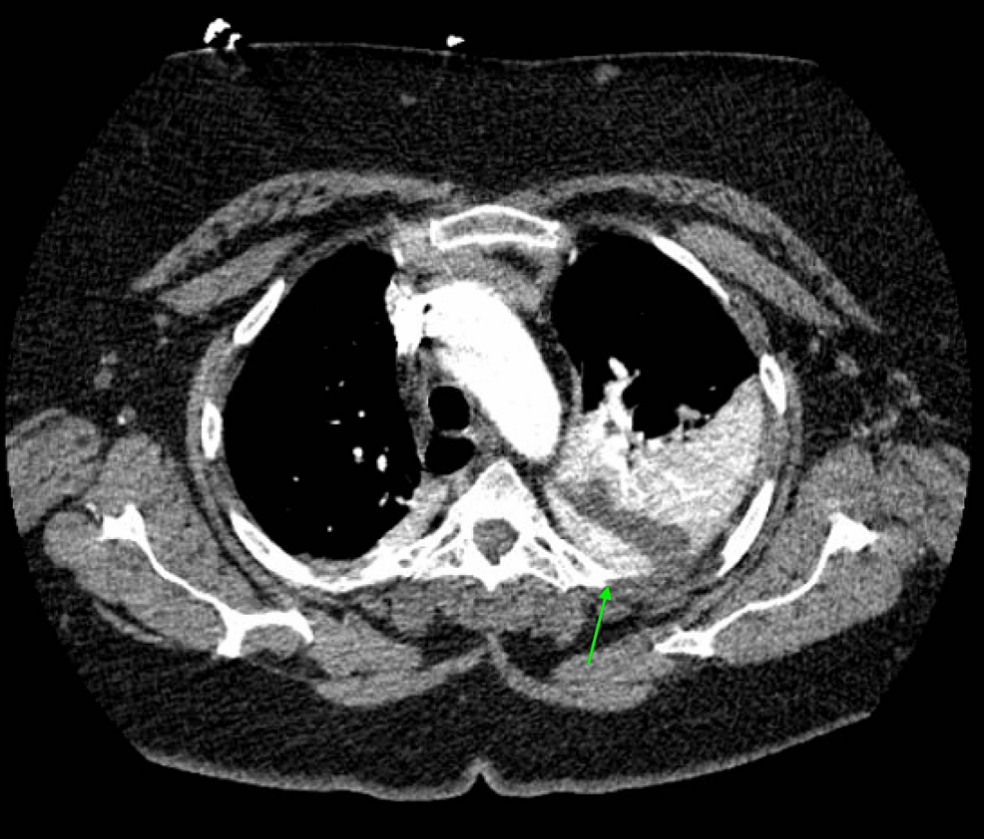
CT PE showing a large left pleural effusion extending across the midline with mass effect causing multifocal atelectasis and trace pneumomediastinum
